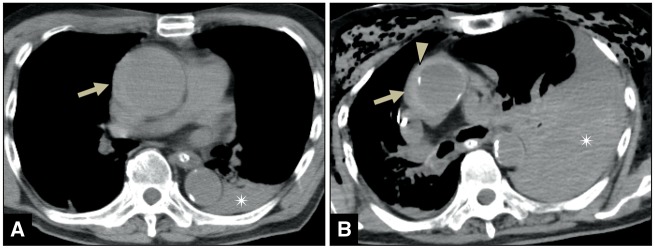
Computed tomography image of Patient 2 after return of spontaneous circulation (ROSC) on the day of admission shows crescentic hyperattenuating intramural fluid collection from the ascending aorta to the arch (*A*-arrow) and left pleural haematoma (*A*-asterisk). Computed tomography image after discontinuation of cardiopulmonary resuscitation on Day 2 of admission shows inward shift of the calcified intima (*B*-arrowhead), significant increase in the left pleural haematoma (*B*-asterisk), and crescentic intramural fluid collection revealing more hyperattenuation (*B*-arrow). The computed tomography values of left pleural haematoma were 33–43 HUs on admission and 45–60 HUs on Day 2 of admission.
